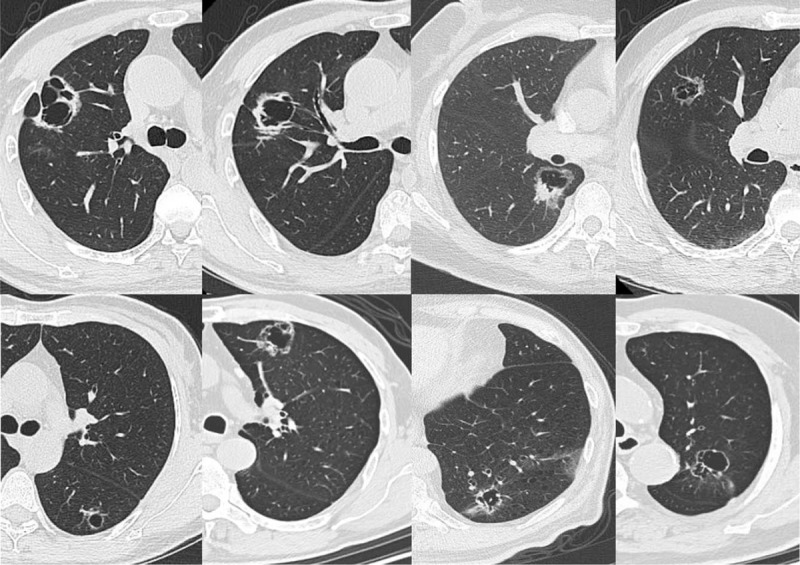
Chest computed tomography presentations of solitary thin-wall cystic lung cancer.
